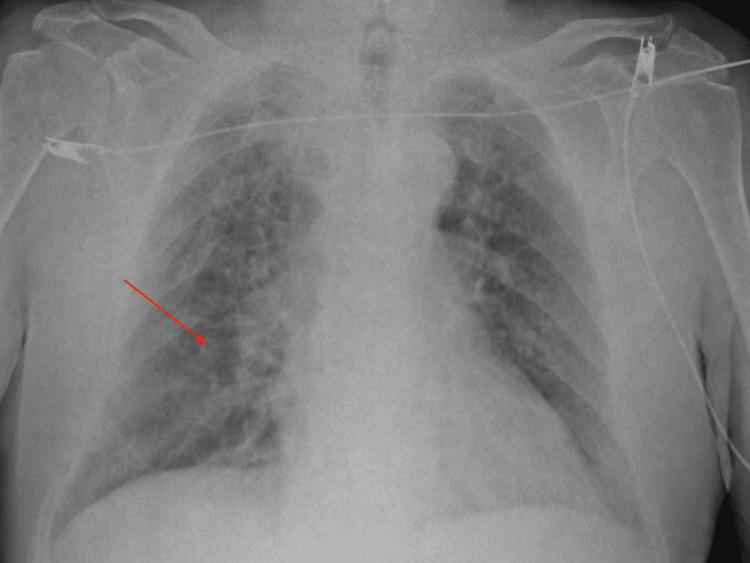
Chest radiograph consistent with cardiac failure Portable anteroposterior chest radiograph showing features in keeping with cardiac failure, including pulmonary vascular congestion/interstitial oedema. The red arrow indicates the perihilar/lower-zone pulmonary congestion, representing the principal radiographic area of interest
